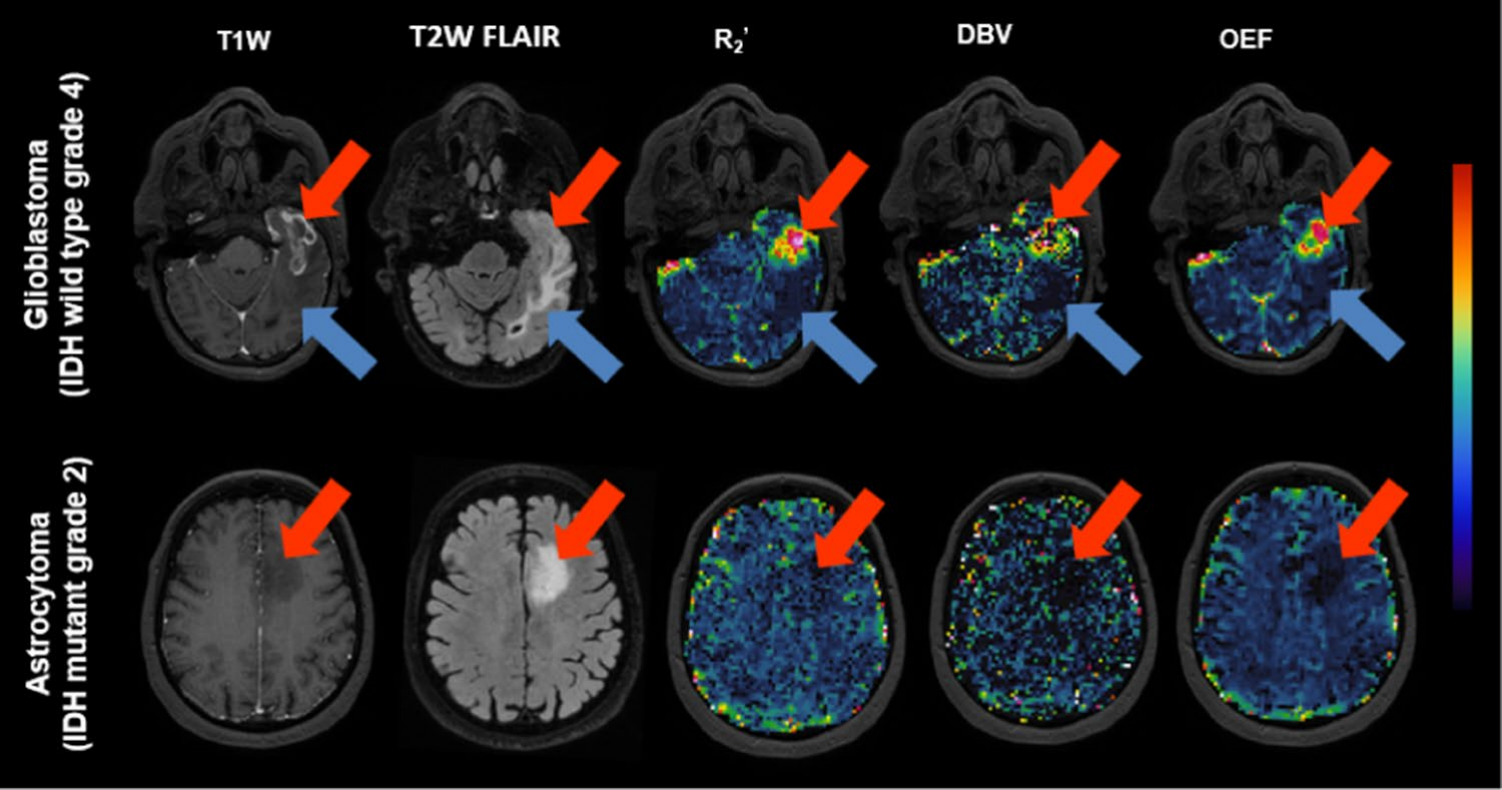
Example slices of two patients with glioblastoma (grade 4) and astrocytoma (grade 2). The images from left to right are, respectively: post-contrast T1W, T2W FLAIR, *R*_2_^'^ (0–40 $${\mathrm{s}}^{-1}$$), DBV (0–10%) and OEF (0–1). Arrows indicate different parts of the tumor: in the first patient, the red arrow points to the contrast enhancing area and necrotic core of the tumor, while the blue arrow points to the surrounding edema. In the second patient, the red arrow points to the tumor area
